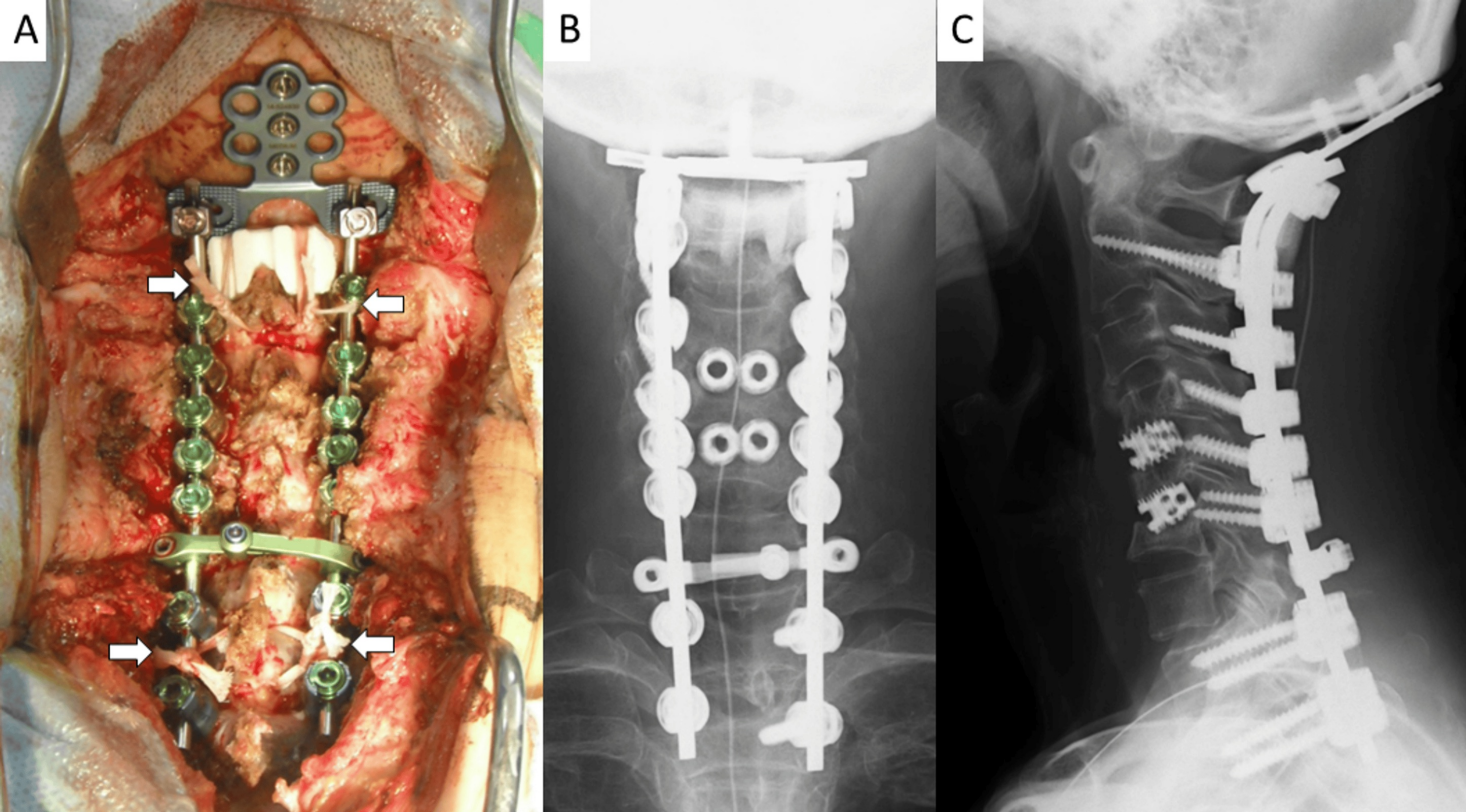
Intraoperative and postoperative images A: Intraoperative photograph showing occiput to T2 posterior fixation. The upper and lower ends of the rods are secured using Nesplon tape (arrows); B: Postoperative anteroposterior radiograph; C: Lateral radiograph demonstrating correction of cervical kyphosis following posterior fixation.
